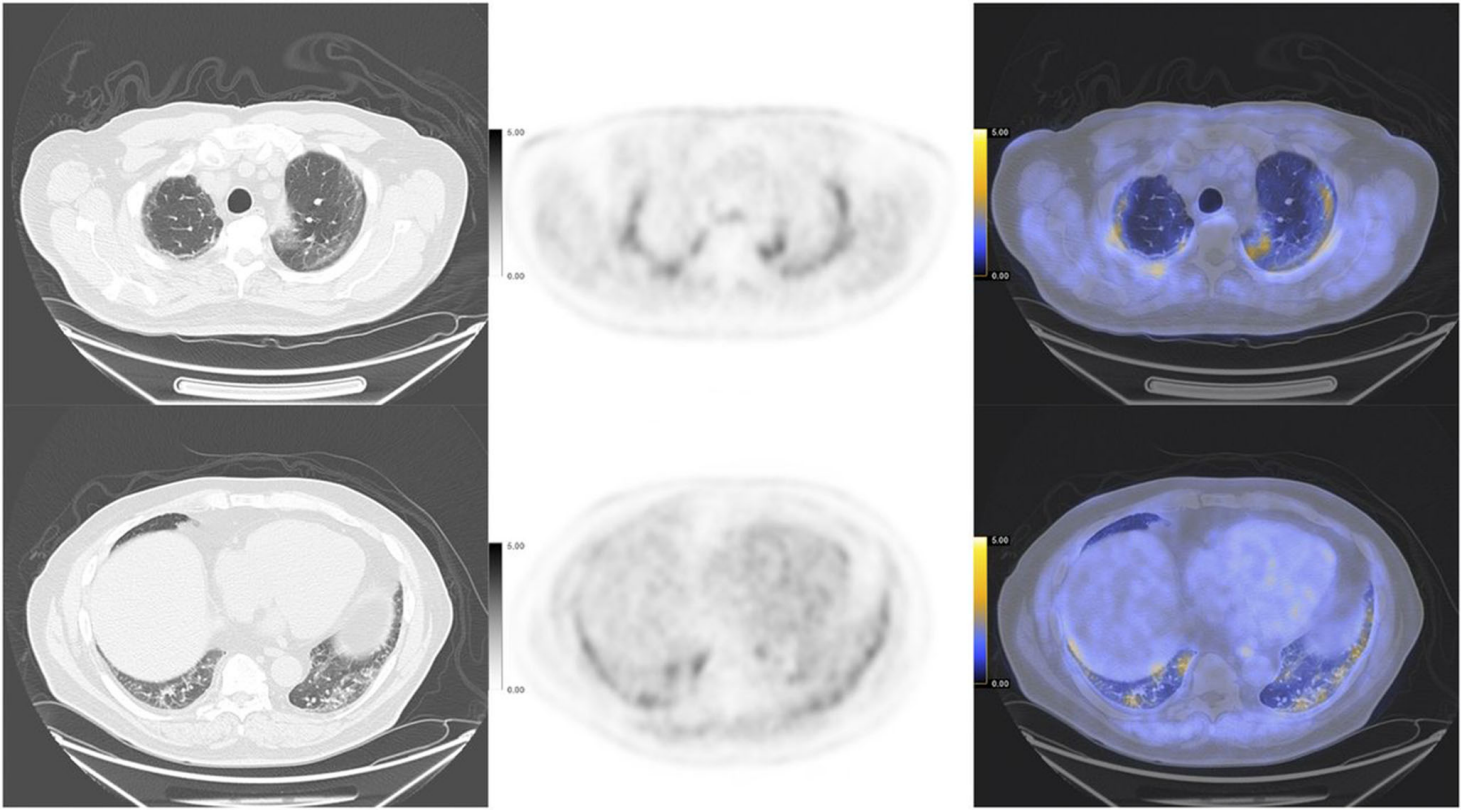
^18^F-αvβ6-BP PET/CT images of a COVID-19-recovered patient 2 months after infection. Axial CT (left), ^18^F-αvβ6-BP PET (middle), and fused PET/CT (right) images showed increased ^18^F-αvβ6-BP uptake (SUVmax 5) and bilateral patchy opacity (reproduced by permission from reference [91])
